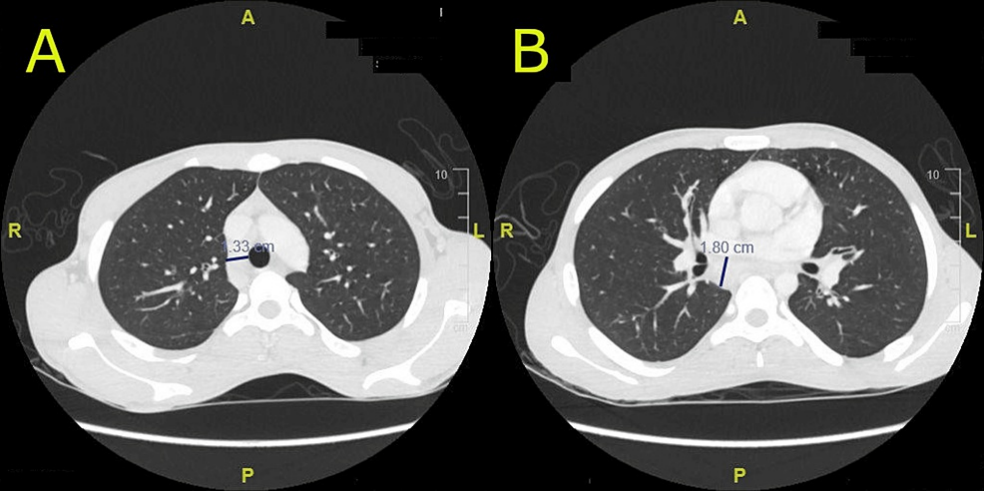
CT scan of the chest A: Axial CT image showing enlarged mediastinal lymph nodes, with the right paratracheal lymph node measuring 1.33 cm. B: Axial CT image showing enlarged mediastinal lymph nodes, the largest of which is in the subcarinal region measuring 1.8 cm. CT: computed tomography
